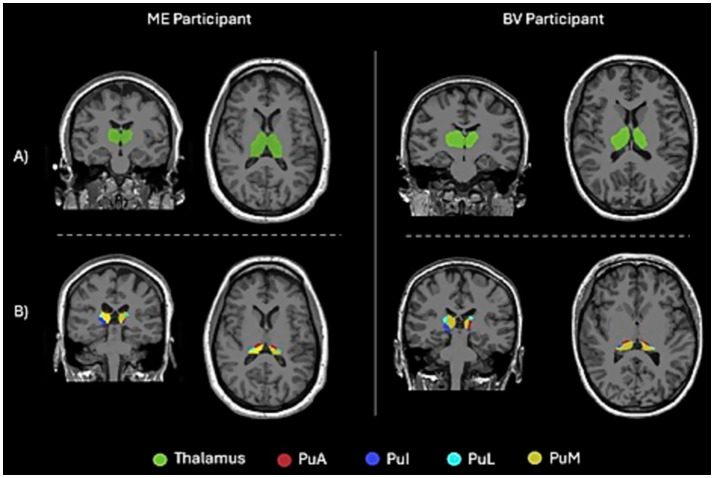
FreeSurfer segmentation of the thalamus and pulvinar in one representative ME participant (left column) and BV control participant (right column). Coronal and axial T1-weighted images are shown. (A) Whole-thalamic segmentation (green), including the pulvinar subdivisions. (B) Whole-pulvinar mask including the anterior (PuA; red), inferior (PuI; dark blue), lateral (PuL; light blue), and medial (PuM; yellow) pulvinar nuclei within each hemisphere. Images are displayed in neurological orientation.
